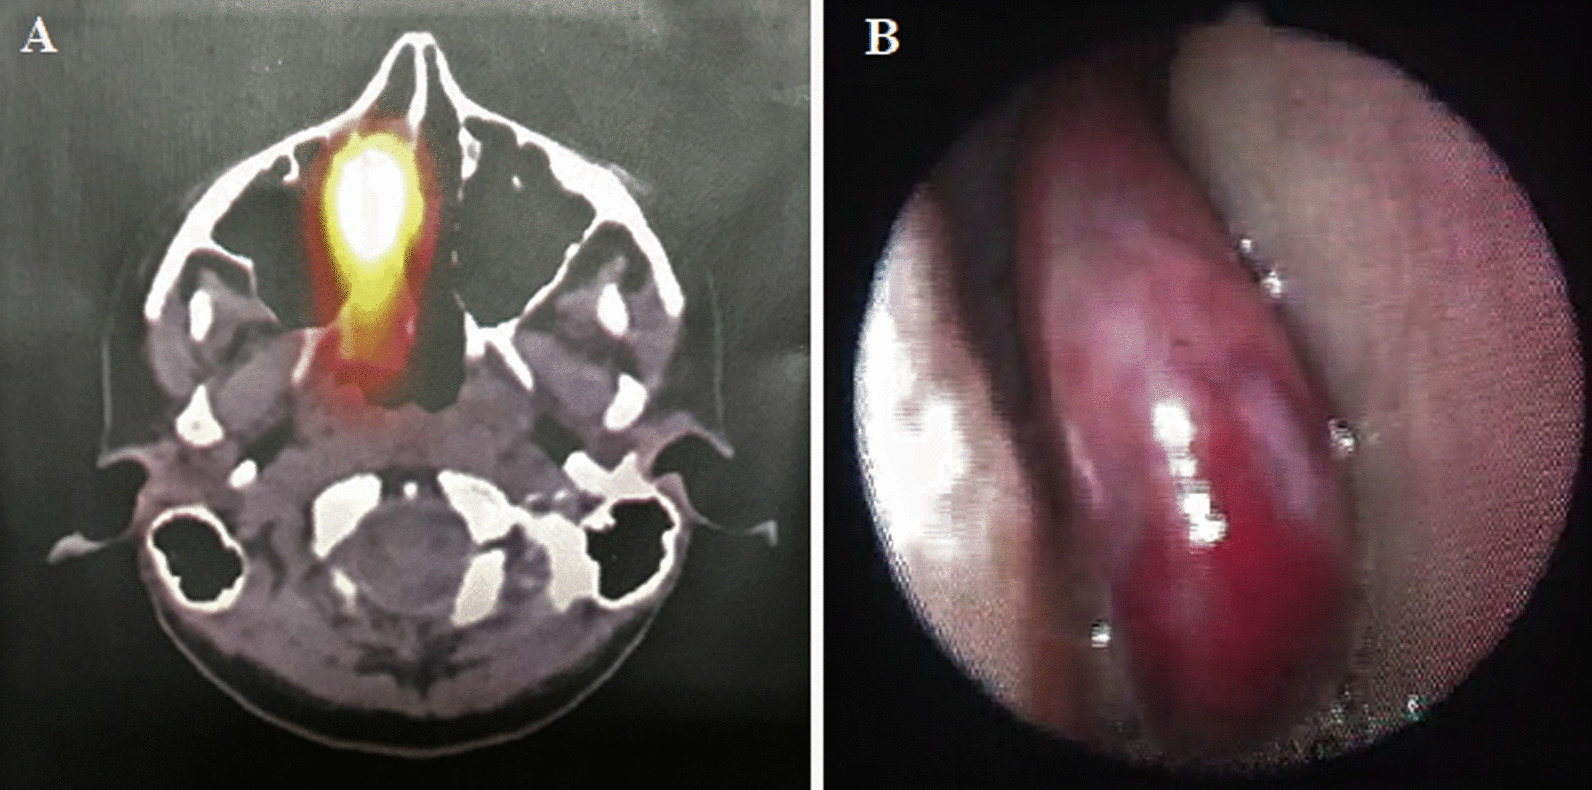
A Somatostatin analog scintigraphy: asymmetric increase of the radiopharmaceutical concentration in the right nasopharynx; B nasofibroscopy: reddish lesion occupying the right nasal cavity from the sphenoethmoidal recess
